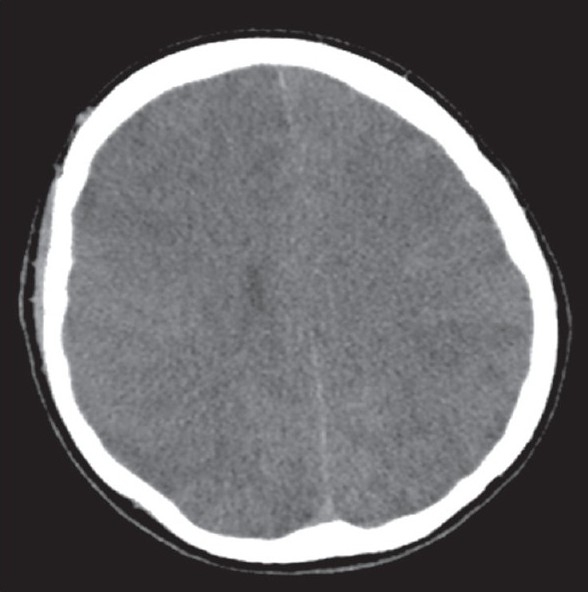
Repeat CT head showing diffuse cerebral edema but no residual intracerebral air
